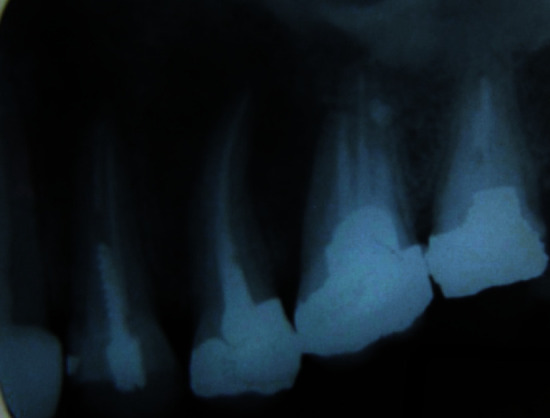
The periapical radiography view: the severe damage was seen in the tooth 24 and 25 region.
